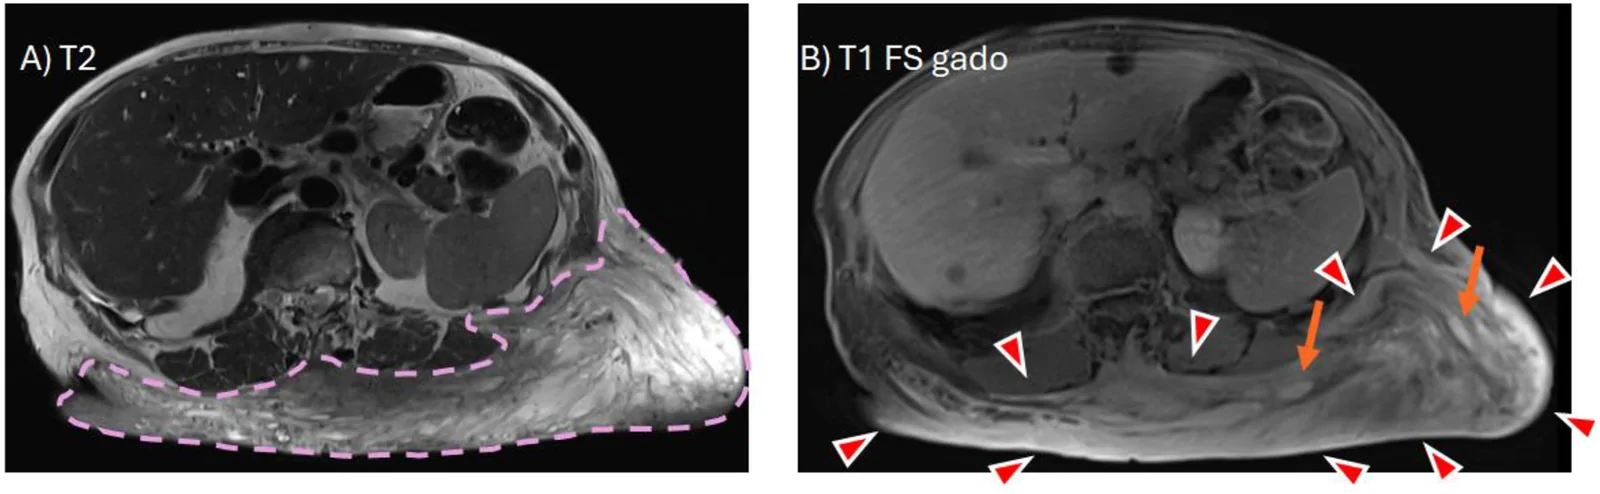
T2-weighed axial MRI view demonstrating extended, diffuse and infiltrative growing of a subcutaneous mass compatible with neurofibroma involving dorsum and left flank with contralateral extension to the right dorsal region (interrupted pink line) (A). T1-weighted axial MRI view with intravenous gadolinium contrast media showing heterogeneous subcutaneous contrast enhancement of the soft tissue mass (red arrowheads), without evidence of intra-abdominal extension. Presence of enhancing nodules within the soft-tissue mass, compatible with neurofibromatous foci (orange arrows) (B).
